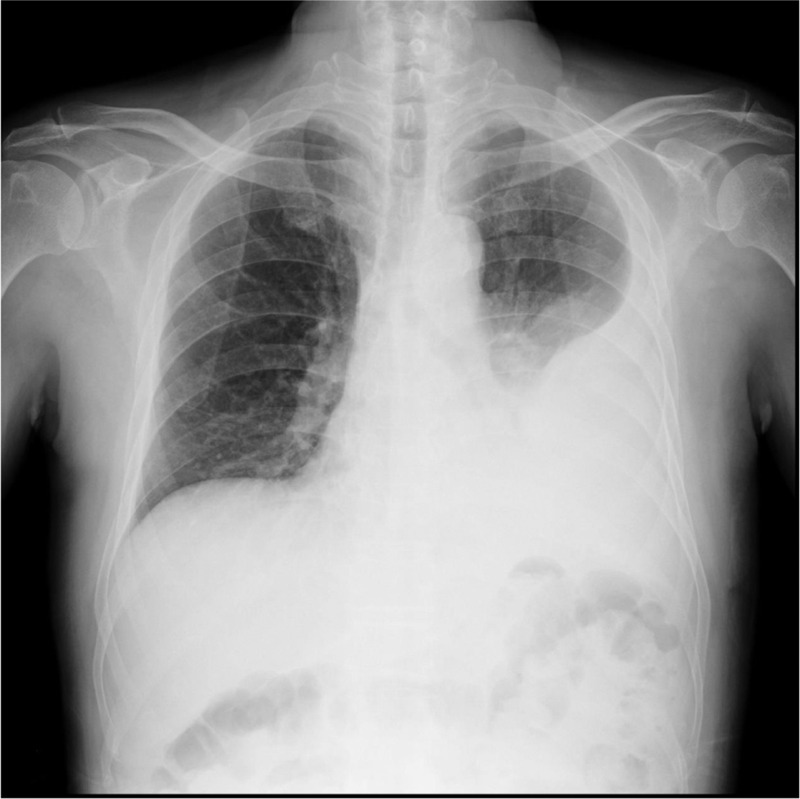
Admission chest radiograph: left lower lung consolidation and pleural effusion.
